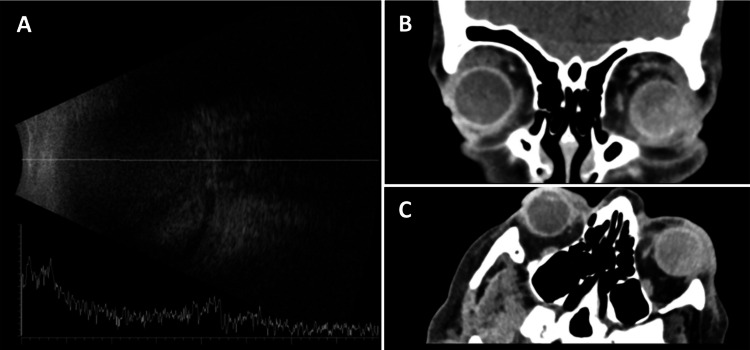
B-scan ultrasonography of the left eye and computerized tomography scans. B-scan ultrasonography of the left eye showed a flat retina with dense hyperechogeneity in the vitreous cavity and an absence of the kissing choroidal sign (A). Computerized tomography (CT) scans (B, coronal plane; C, transverse plane) showed hyperdensity in the posterior segment of the patient's left eye.
